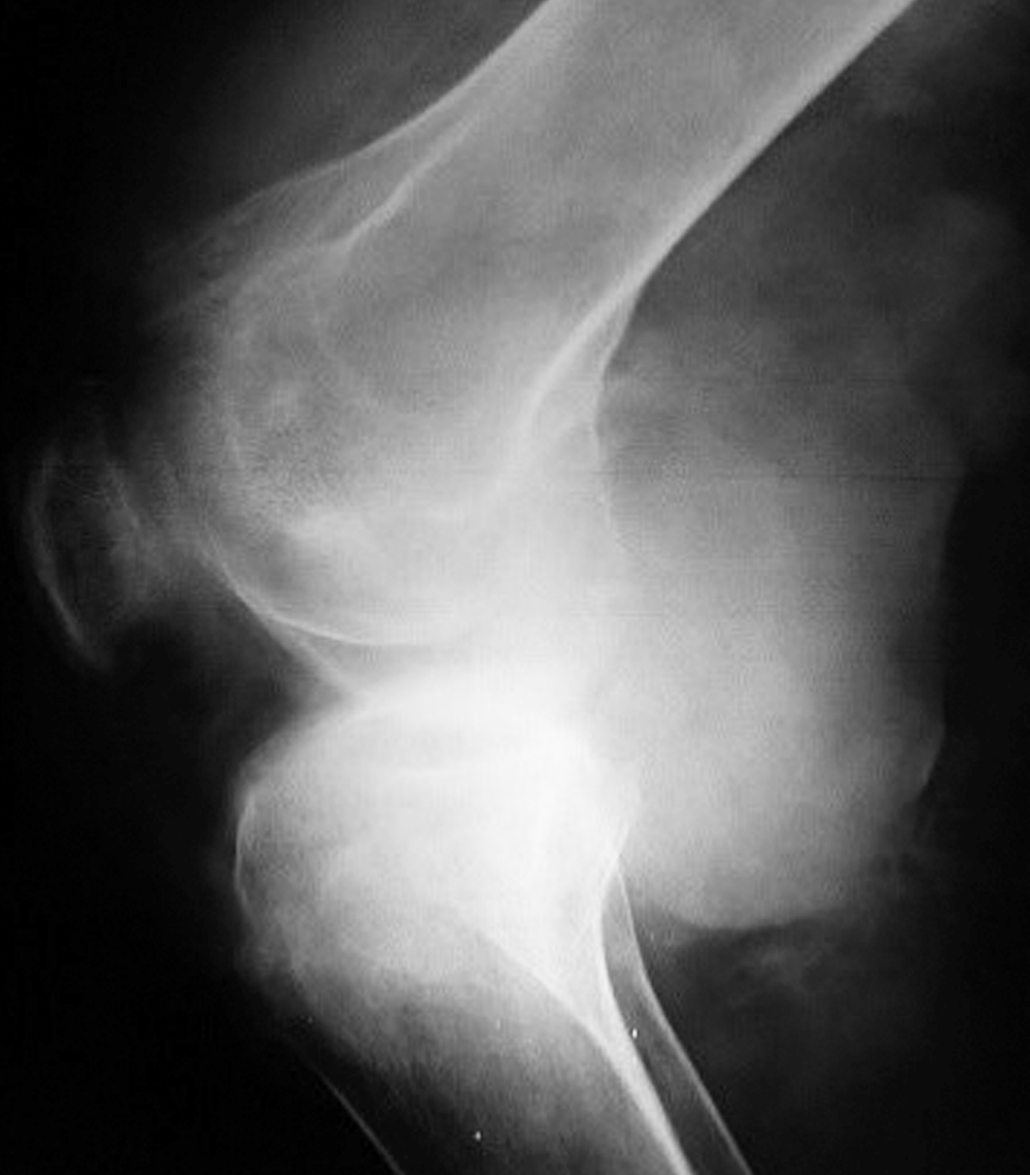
X-ray of the patient’s right knee joint region showing massive soft tissue mass and condylar notch widening with massive effusion.
